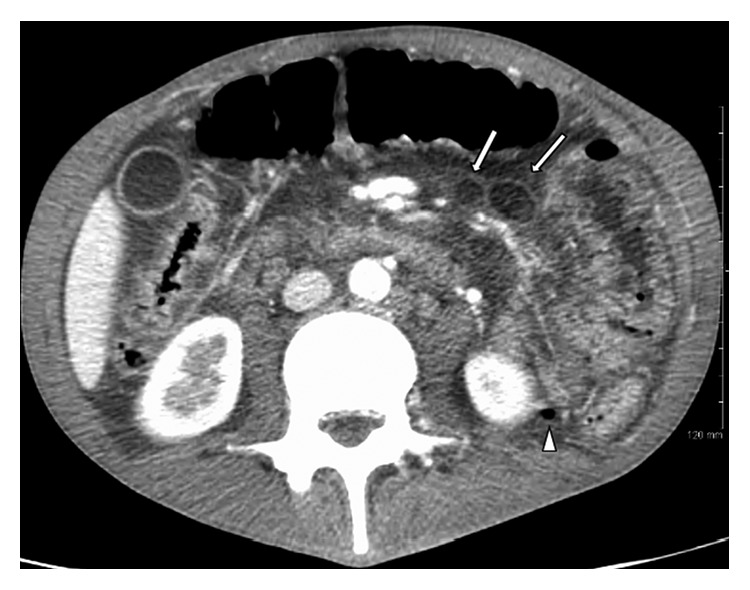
CT abdomen/pelvis with IV contrast (axial). White arrows: mesenteric masses found to be histoplasmosis after surgical resection. White arrowhead: free air in the mesentery from jejunal perforation.
